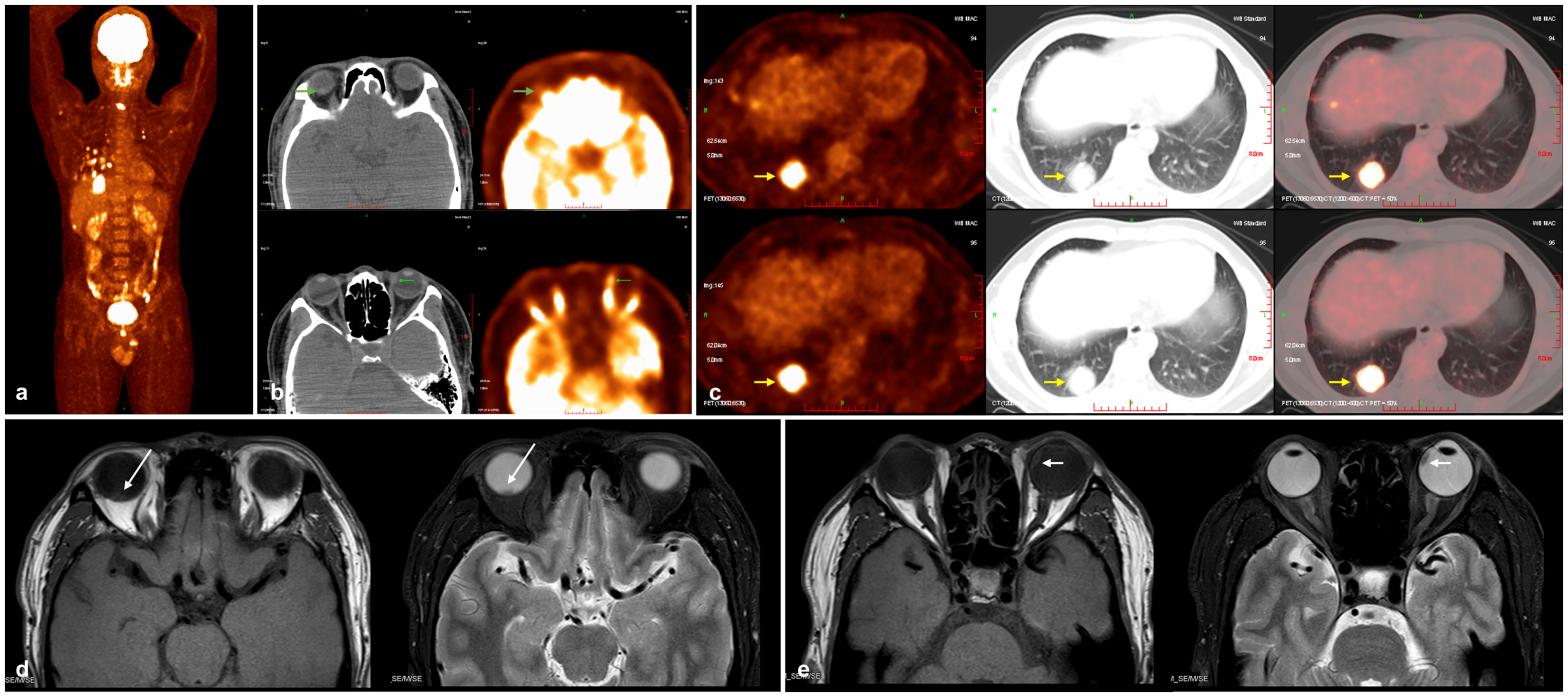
Whole-body FDG PET/CT and orbital MRI scans. (a) FDG PET/CT (maximum intensity projection) demonstrates hypermetabolic lesions in bronchial lymph nodes, skeletal system, and lungs. (b) FDG PET/CT shows increased FDG uptake in the masses (green arrow). (c) The largest pulmonary mass is located in the posterior basal segment of the right lower lobe (yellow arrow). (d,e) MRI scans reveal heterogeneous isointense signals in the lesions of both eyes on T1-weighted images, and heterogeneous hypointense signals on T2-weighted images (right eye indicated by a white long arrow, left eye indicated by a white short arrow). FDG PET/CT, fluorodeoxyglucose positron emission tomography/computed tomography; MRI, magnetic resonance imaging.
